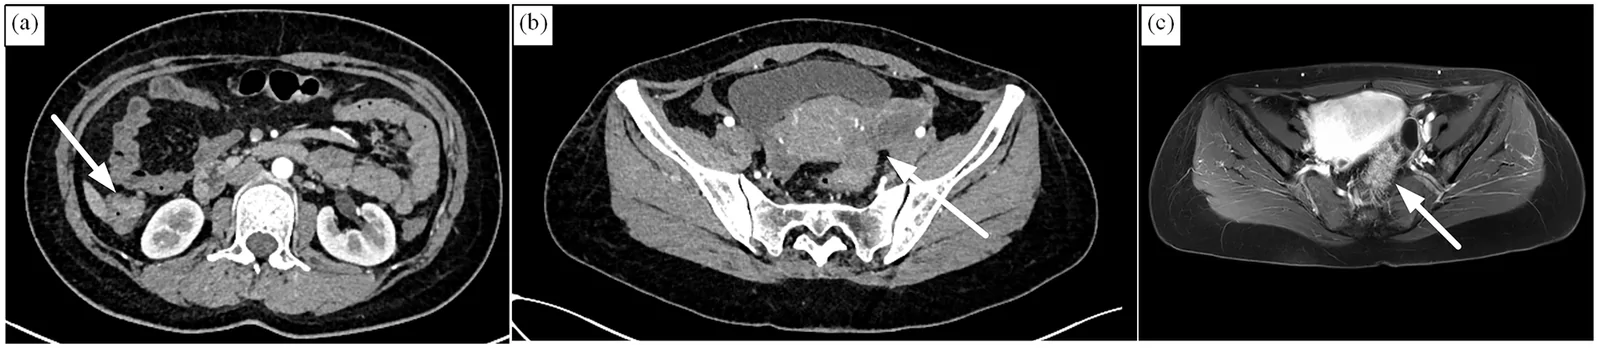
Imaging findings. (a) Contrast-enhanced CT of the ascending colon showing mild wall thickening at the hepatic flexure (white arrow). (b) Contrast-enhanced CT of the sigmoid colon showing circumferential wall thickening (white arrow). (c) Contrast-enhanced MRI demonstrating similar findings in the sigmoid colon with ill-defined borders (white arrow).
